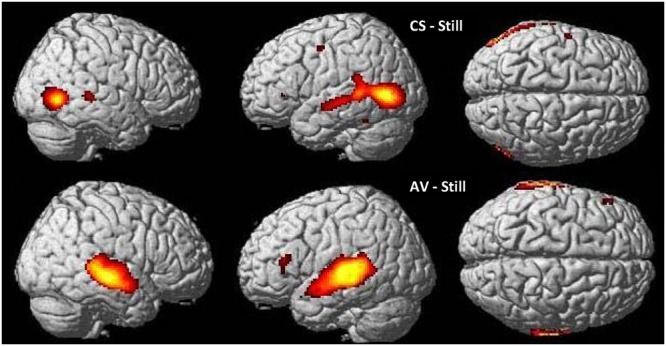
Word perception in CSLM and speech AV. Higher activations during CSLM and speech AV perception of words as compared to the still control condition. All activations are displayed at *p* < 0.05 whole brain corrected, superimposed on the SPM MRI template. CSLM, Cued Speech (oral + manual) in deaf early users; AV, audiovisual speech in hearing speakers.
